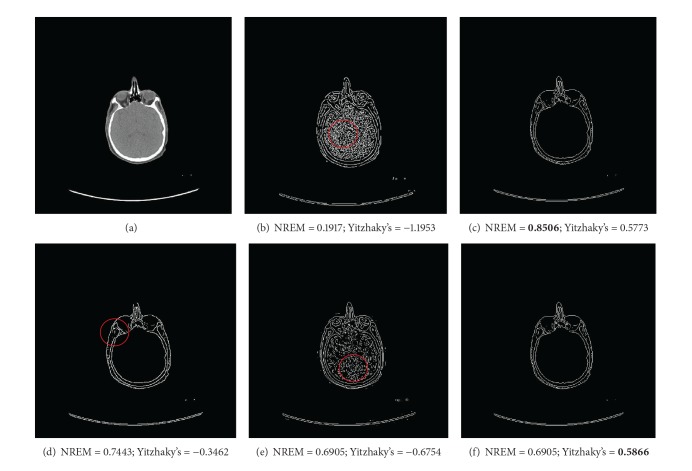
Using measures to select optimal edge detection algorithms. (a) Original CT abdomen image. (b)–(f) Edge detection results from (b) Canny, (c) Sobel, (d) Roberts, (e) LoG, and(f) Prewitt. The optimal edge maps with highest NREM value and Yitzhaky's measure value are in bold.
